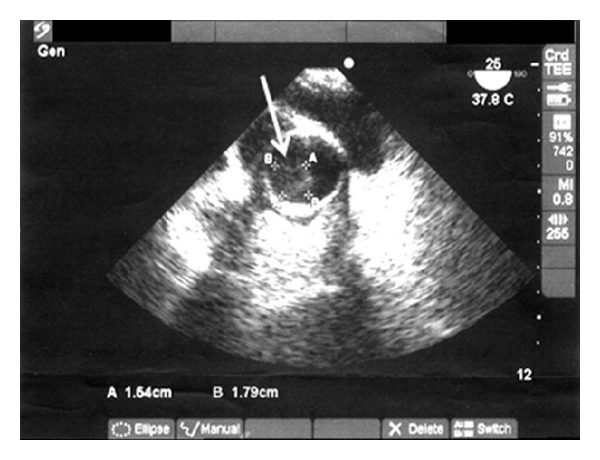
Transesophageal echocardiography with vegetation of aortic graft joint.
